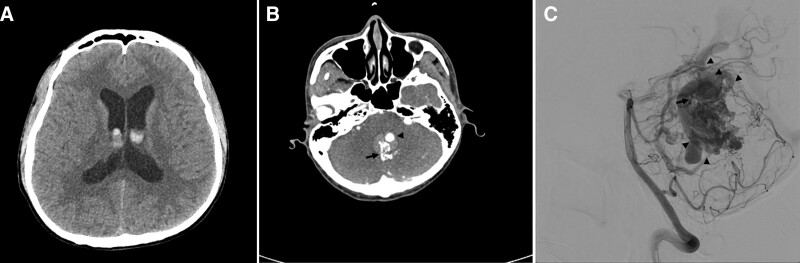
(A) Non-enhanced head computed tomography (CT) demonstrated a small intraventricular hemorrhage with enlarged ventricle. (B) CT angiography revealed an arteriovenous malformation (AVM) nidus (arrow) with dilated draining veins in the cerebellum (arrow head). (C) Lateral projection of the left vertebral artery distal subtraction angiography. The nidus was approximately 3.2 cm in maximum diameter, mainly supplied by the engorged branch of left superior cerebellar artery (SCA) (arrow). Two main dilated venous outlets were observed draining into the vein of Labbé, internal cerebral vein, and vein of Galen with aneurysmal dilatation (arrow head).
